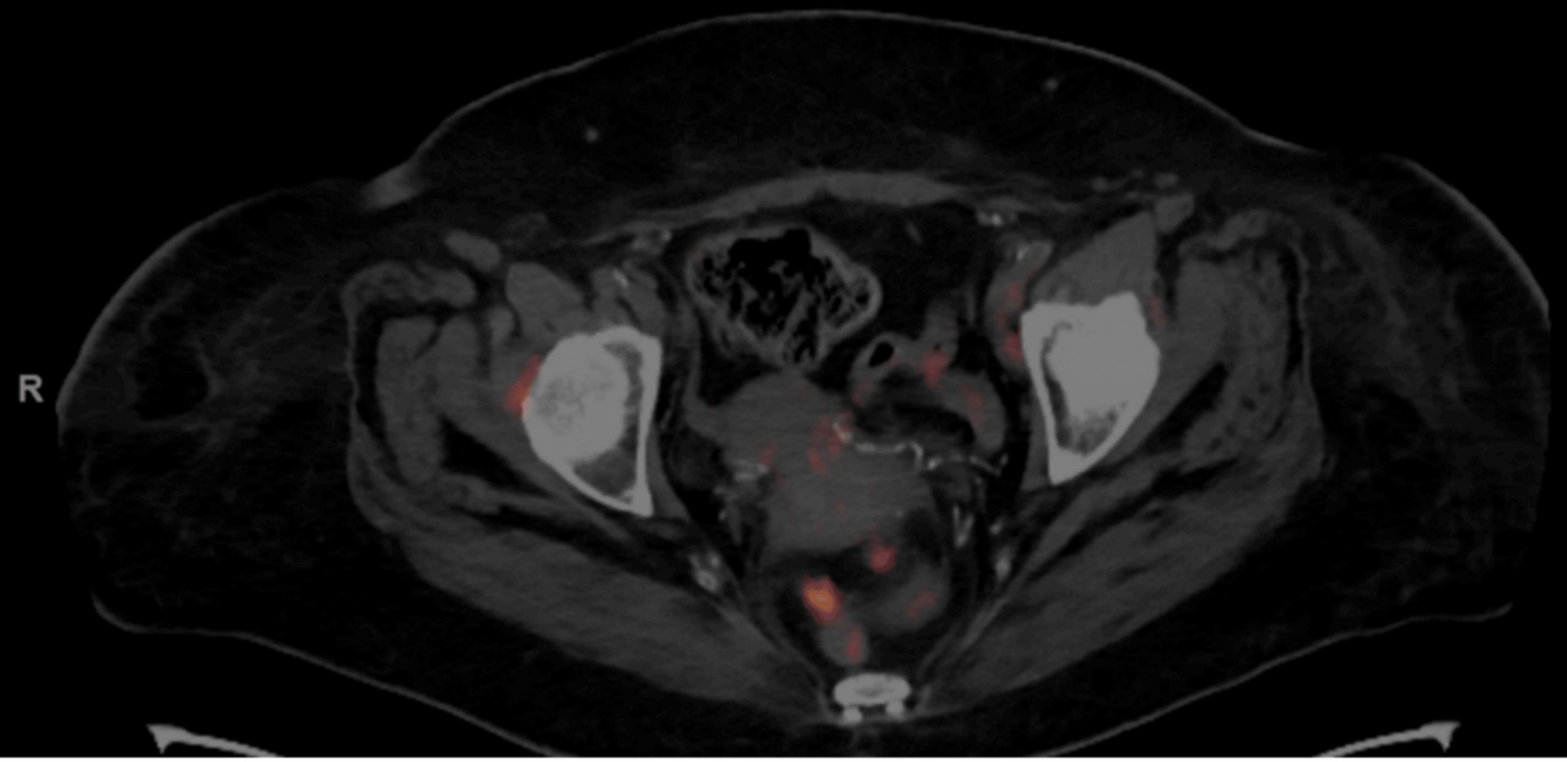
PET-CT scan of the pelvis The image shows two foci of abnormal soft tissue and increased FDG uptake in the cul-de-sac region between the upper rectum and upper vaginal pouch, suspicious for metastatic foci PET-CT: positron emission tomography-computed tomography
